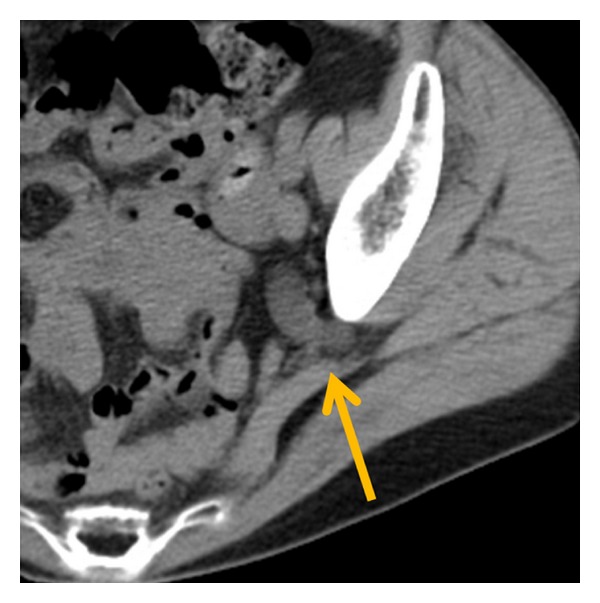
Computed tomography showed ureteral herniation into the sciatic foramen (arrow).
